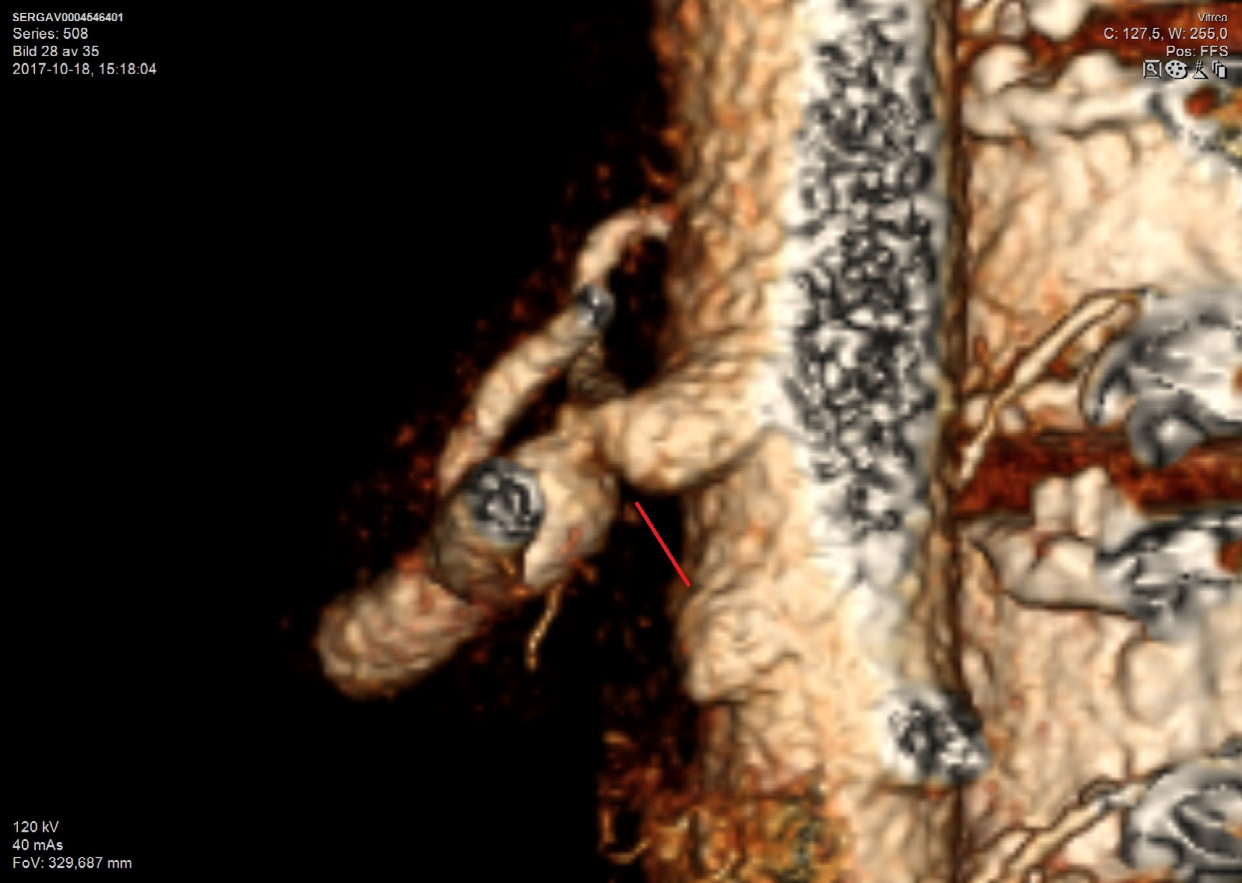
Abdominal computed tomography angiography showing the total occlusion of truncus coeliacus.
